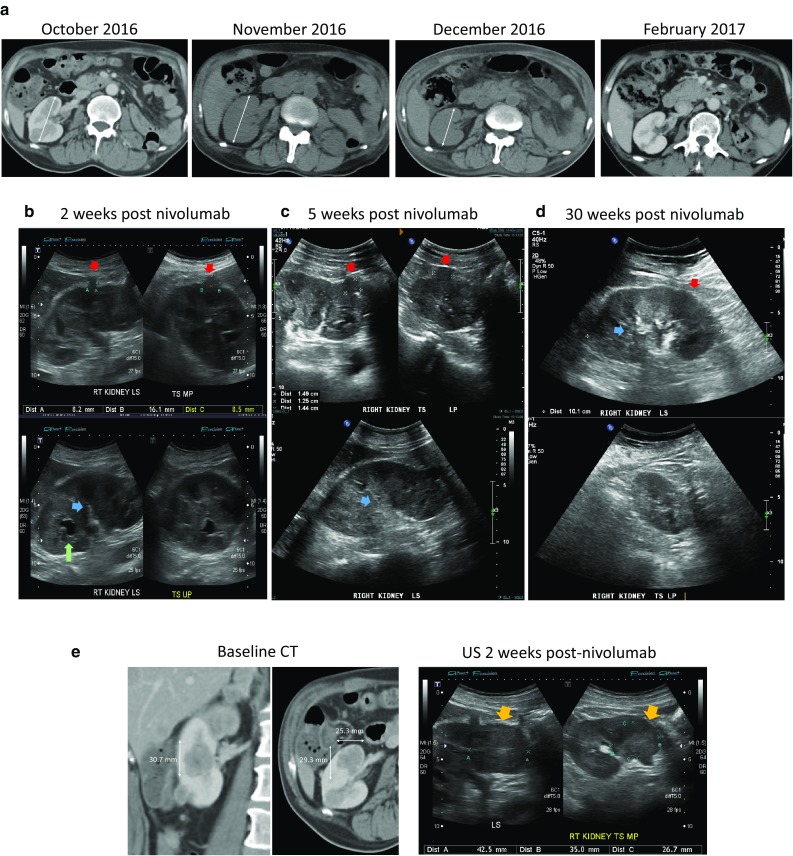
Renal Imaging. a Serial CT images with marginal increase in renal size from baseline (October) to 5 weeks after nivolumab (November), marked decrease in renal size at 11 weeks (December) and complete resolution of intrarenal tumors at 20 weeks (February). The changes correspond to the initial deterioration of renal function after nivolumab administration followed by recovery. b, c Serial US images during the acute renal failure phase after nivolumab. From week 2 to 5 an enlarging tumor is demonstrated (top, red arrows). There is concomitant increase in cortical swelling with compression and obscuration of the renal medulla and sinus fat (bottom, blue arrows). A renal calyx (bottom, green arrow) seen at week 2 is also subsequently obscured. d Corresponding renal US images at week 30, with resolution of renal metastases and cortical swelling, and normal appearance of renal medulla and sinus fat. e Increase in a lower pole tumor from baseline CT to the US done at 2 weeks post-nivolumab (yellow arrows)
